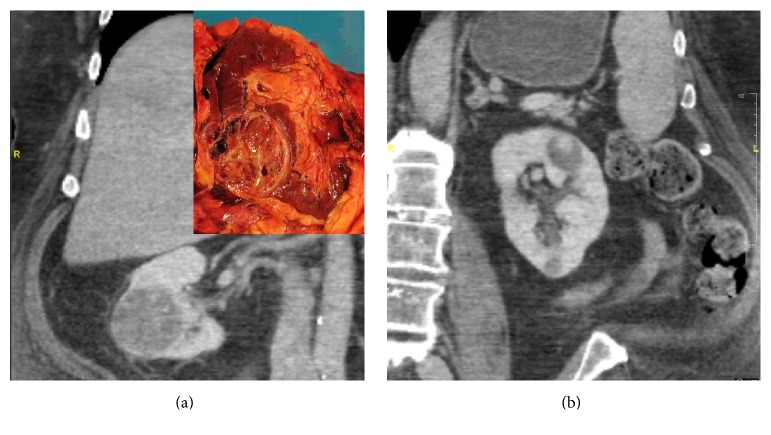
Axial CT images of the (a) right kidney with the largest mid-kidney mass and corresponding gross image [inset] and (b) left kidney with largest upper pole mass.
